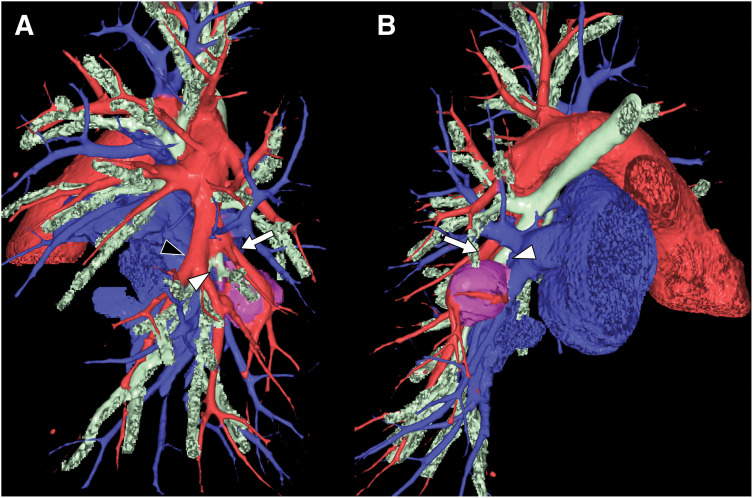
Preoperative 3D-CT. (A) Interlobar view: A10 (white arrow) is clearly visible and the bifurcation of B10 (white arrowhead) can be exposed after transection of A10 and retraction of A8+9 (black arrowhead). (B) Posterior view: Due to the tumor location, A10 (white arrow) and the B10 bifurcation (white arrowhead) are not visible.
